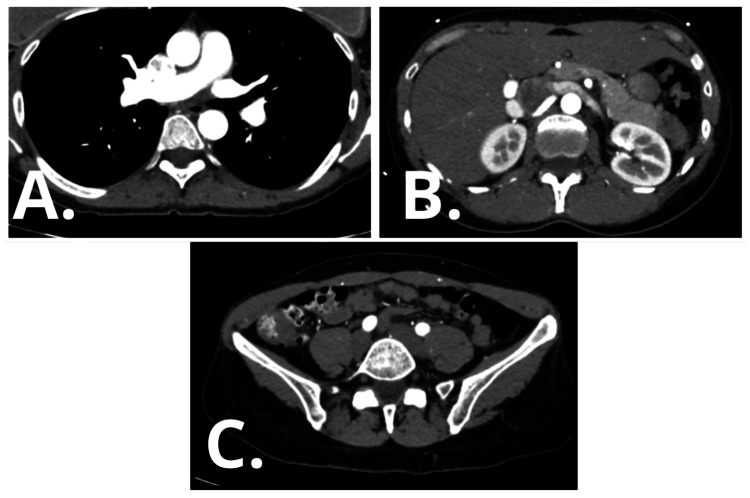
Computed tomography angiography of the thoracic, abdominal, and pelvic vasculature performed to evaluate for associated arteriopathies (A) Thoracic view demonstrating a normal thoracic aorta without aneurysm or dissection. (B) Abdominal view showing normal renal arteries without stenosis or "string-of-beads" appearance suggestive of fibromuscular dysplasia. (C) Pelvic view demonstrating normal iliac arteries. No vascular abnormalities were identified.
